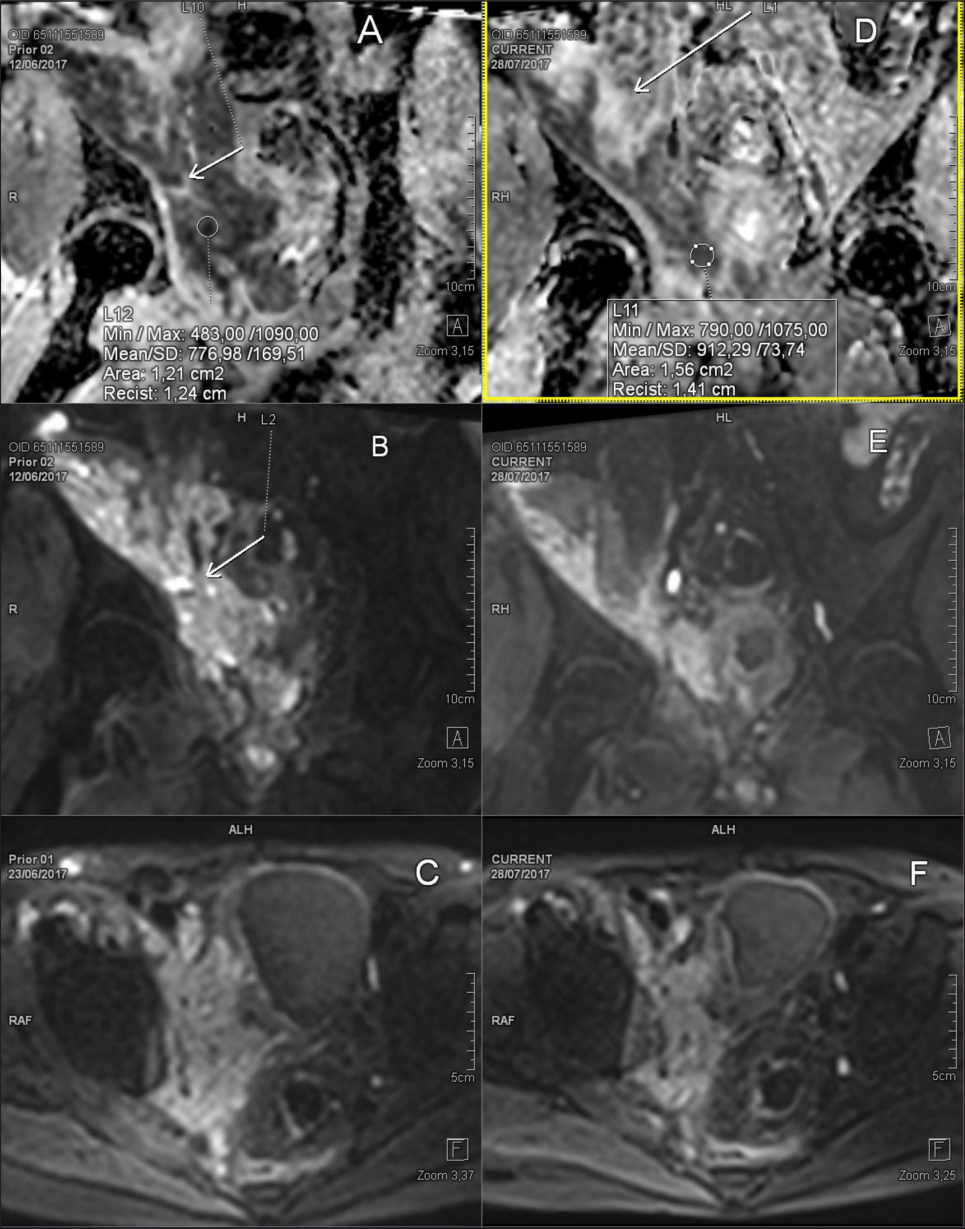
DWI images at initial presentation (A, B, C) and after six weeks of antibiotics (D, E, F).
